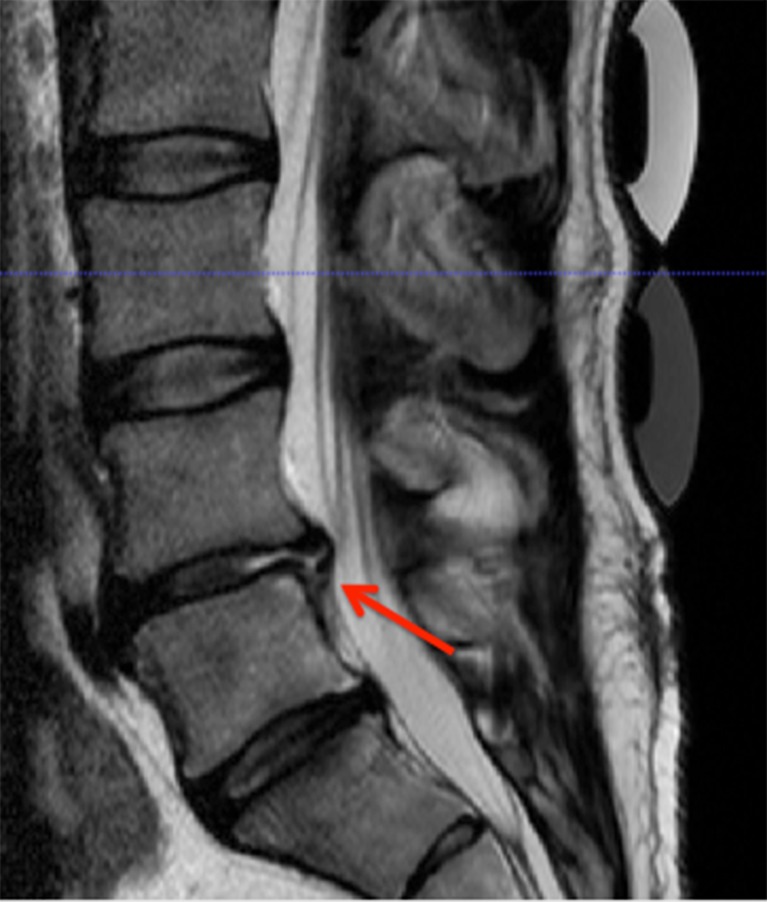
Post-transplant T2-weighted MRI. An extradiscal fluid collection (➔) was observed in three patients after transplantation surgery
